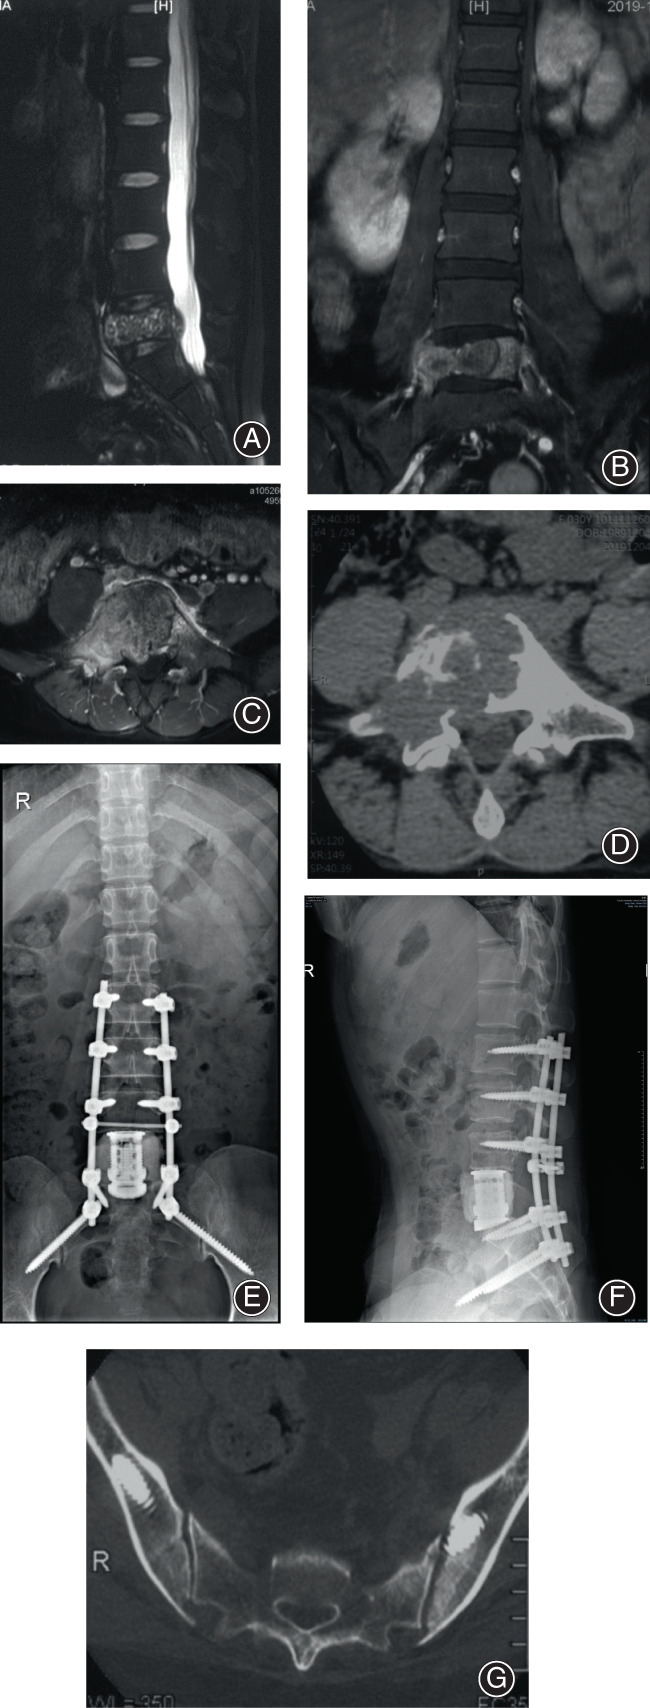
Case 15. Giant cell tumor of bone at L5 in a 30‐year‐old woman. (A) Sagittal T2‐weighted magnetic resonance imaging showed the tumor involving L5 vertebral body. Coronal (B) and axial (C) T1‐weighted enhanced magnetic resonance imaging demonstrated the extent of the tumor with spinal canal compromise. (D) Axial computed tomographic scan demonstrated the tumor with osteolytic destruction. Anteroposterior (E) and lateral (F) radiographs showed a stable construct at 2 years postoperatively. (G) Postoperative computed tomographic scan demonstrated no breach of the screws
